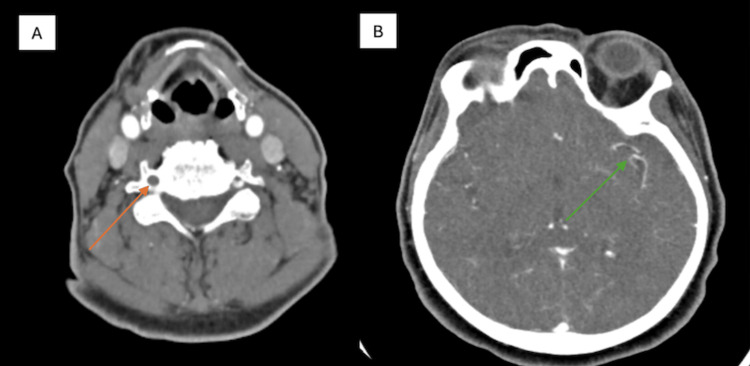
Axial CT angiography of the head and neck. (A) Right vertebral artery dissection at the level of C3 (orange arrow), with a patent left vertebral artery and preserved blood flow. (B) 3.5 mm saccular aneurysm at the bifurcation of the left middle cerebral artery (MCA) (green arrow). CT = computed tomography; C3 = third cervical vertebra; MCA = middle cerebral artery.
